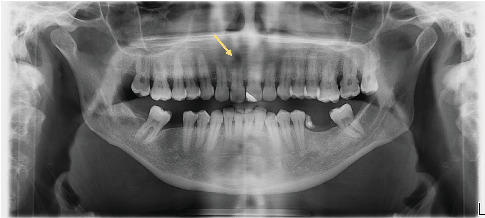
Panoramic X-ray: radiolucent periapical image on the right maxillary central incisor (yellow arrow) and the absence of any notable abnormalities on the left side that could explain the clinical presentation.
